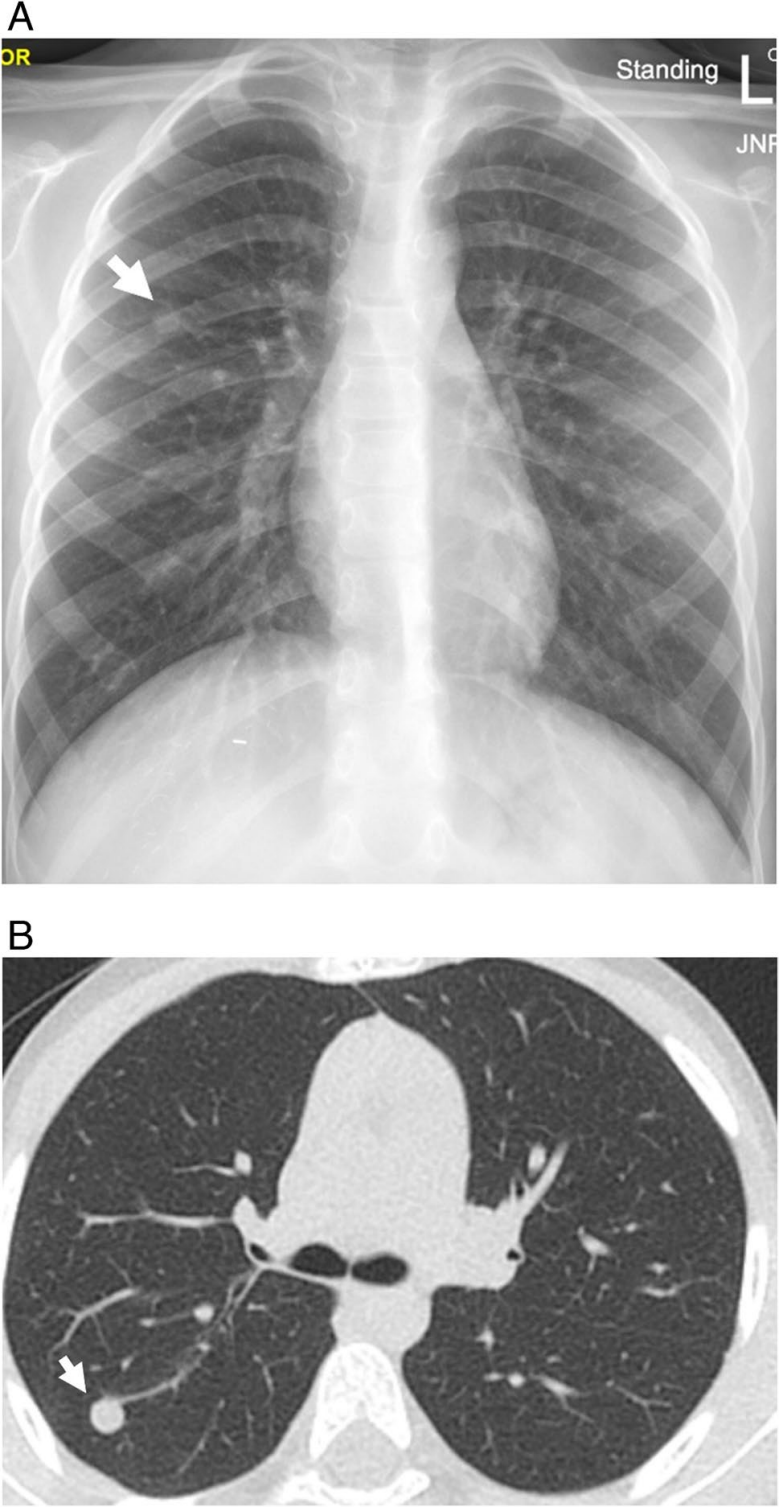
Mucoepidermoid carcinoma in a 6-year-old male with history of hepatoblastoma status post hepatic resection and chemotherapy 5 years prior. A Screening chest radiograph demonstrates a nodular opacity in the right upper lobe (*arrow*). B Non-contrast chest CT image shows a corresponding round, well defined right upper lobe nodule (*arrow*)
